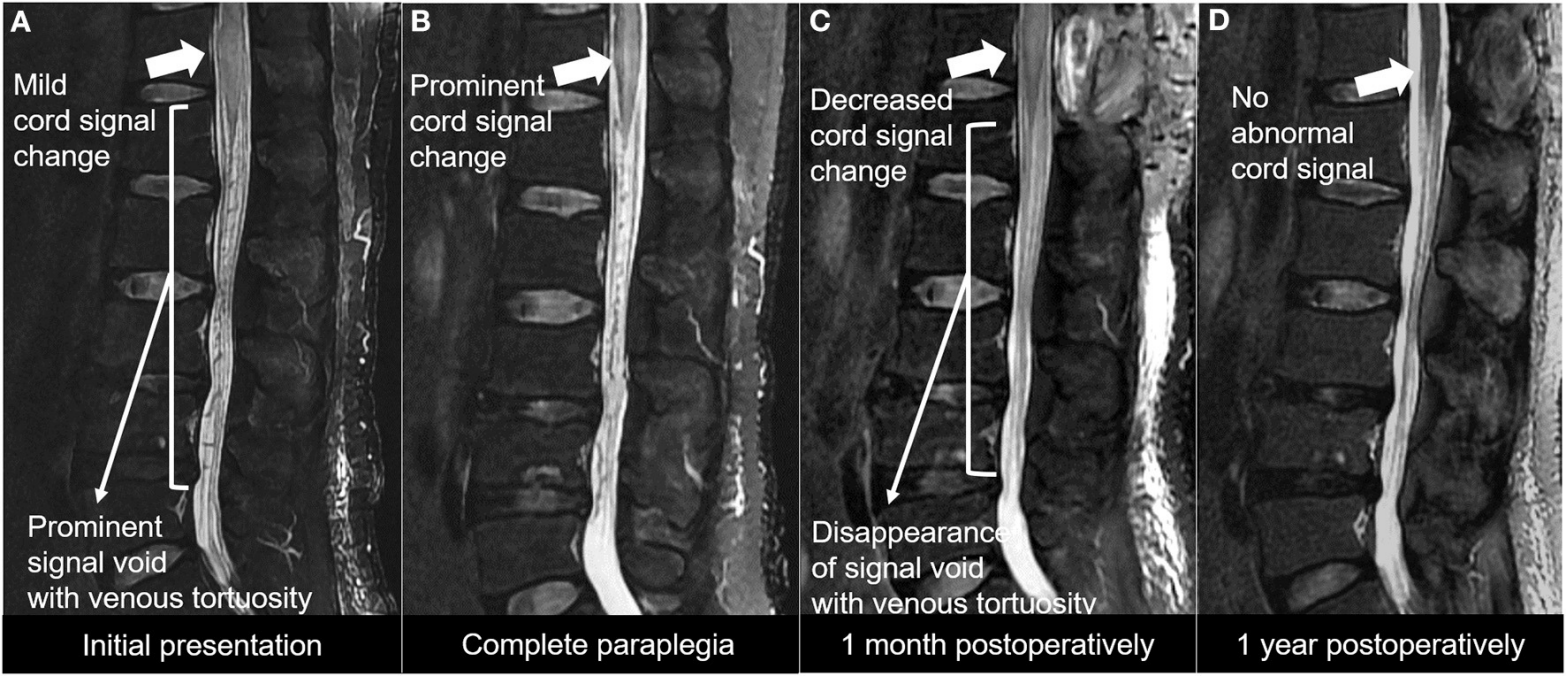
(A) T2-weighted MRI study showing prominent signal void with redundant venous tortuosity, which represents spinal arteriovenous fistula. (B) T2-weighted MRI study showing worsening of spinal cord signal change. (C) T2-weighted MRI study at post-operative 1 month showing decreased cord signal change and disappearance of the signal void with redundant venous tortuosity. (D) T2-weighted MRI study at post-operative 1 year no abnormal cord signal change.
